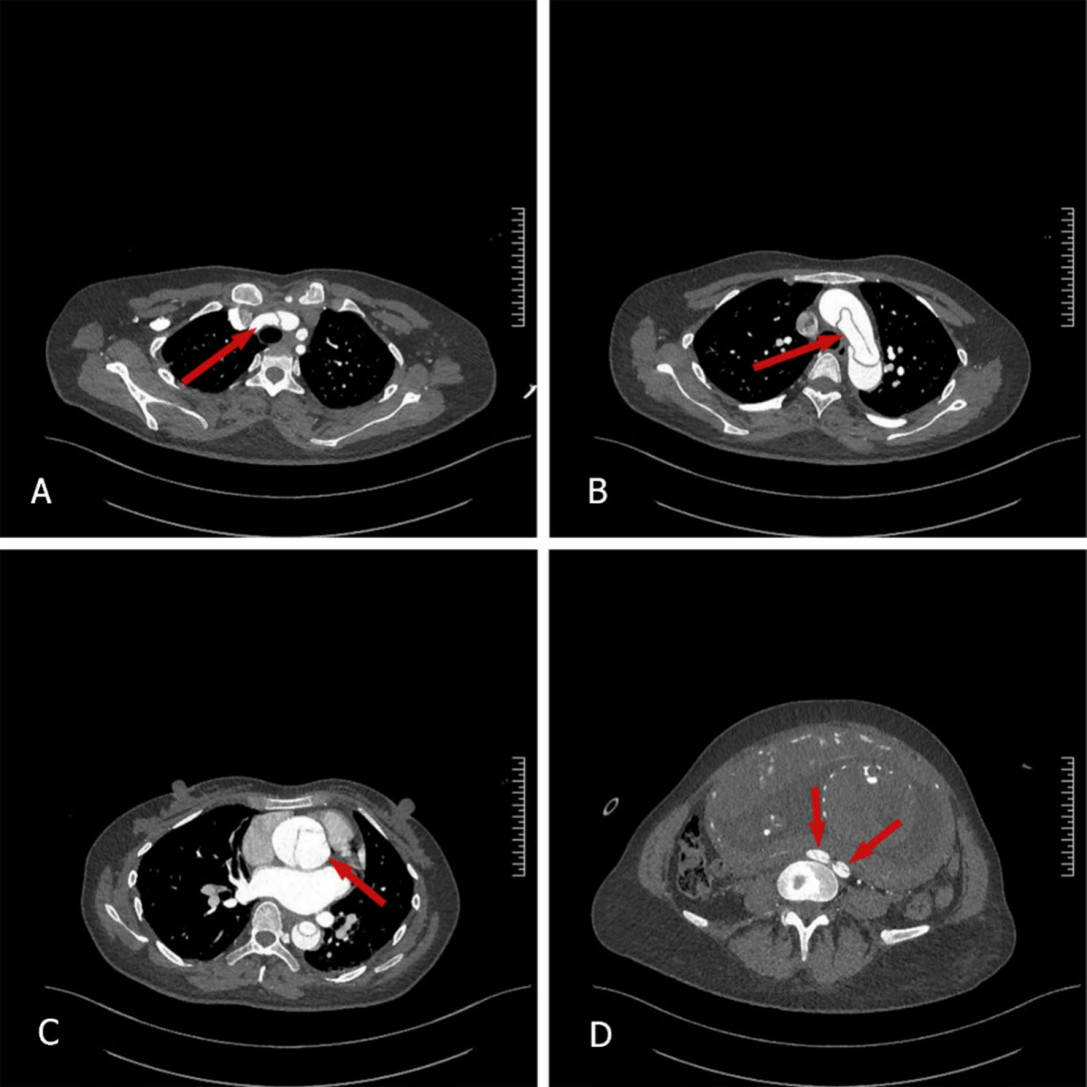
Axial computed tomography images of a 40-year-old woman with acute type A aortic dissection at 31 weeks of gestation, located at the levels of (A) the brachiocephalic trunk, (B) the aortic arch, (C) the ascending aorta, and (D) the iliac bifurcation
